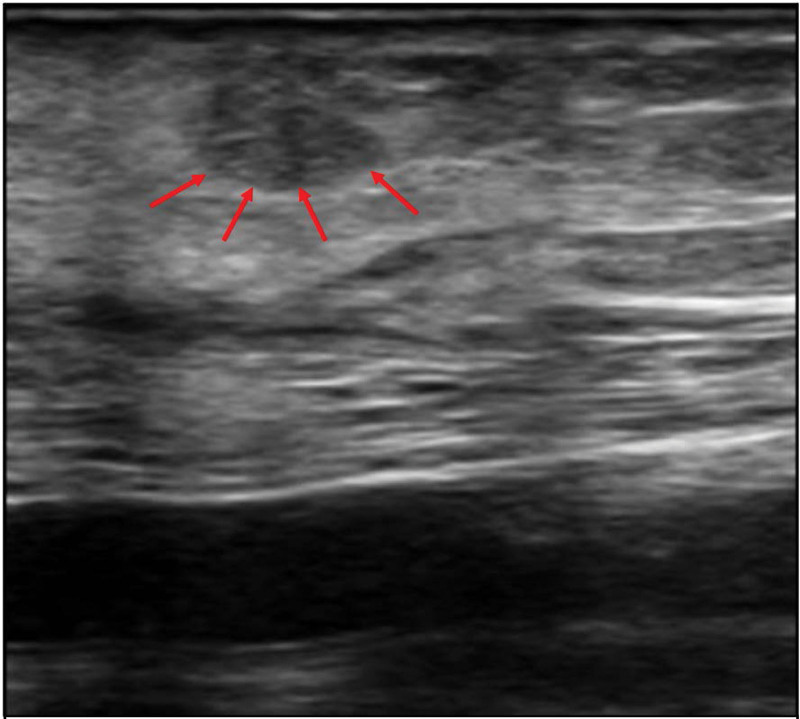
Intraoperative ultrasonography at secondary operation showed a hypoechoic lesion (2 cm × 1.4 cm × 0.6 cm, arrow), which was 3 times of initial size. (figure 1. Arrow).
